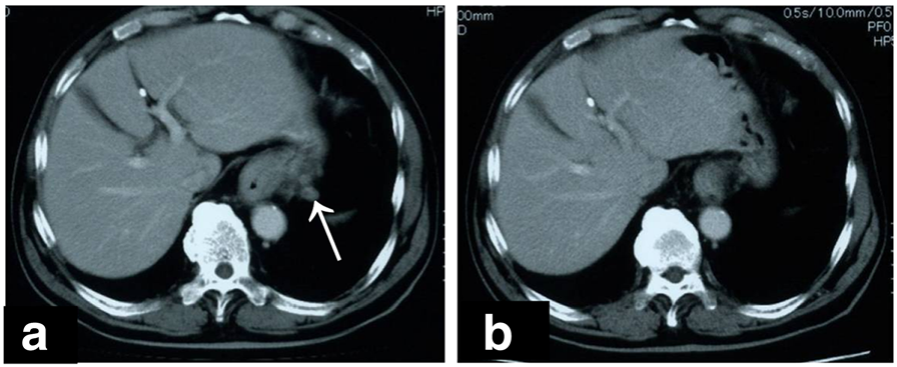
Computed tomography images before and after treatment. Initial computed tomography revealed multiple lymph node metastases around esophagus. (b) Follow-up computed tomography revealed that multiple lymph node metastases around esophagus had completely disappeared.
